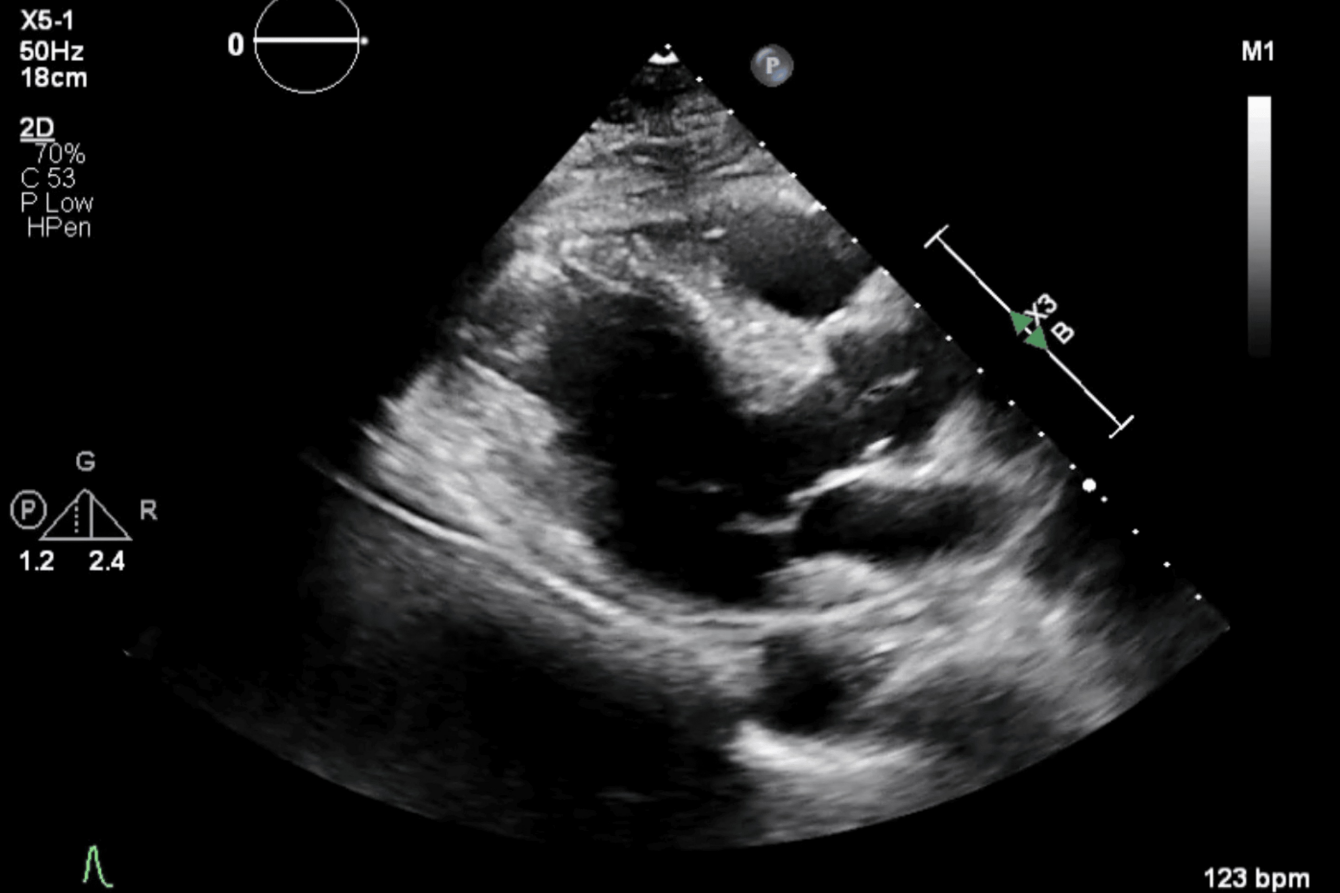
Post-procedural 2D echo with severely reduced EF. Echo: echocardiogram; EF: ejection fraction
